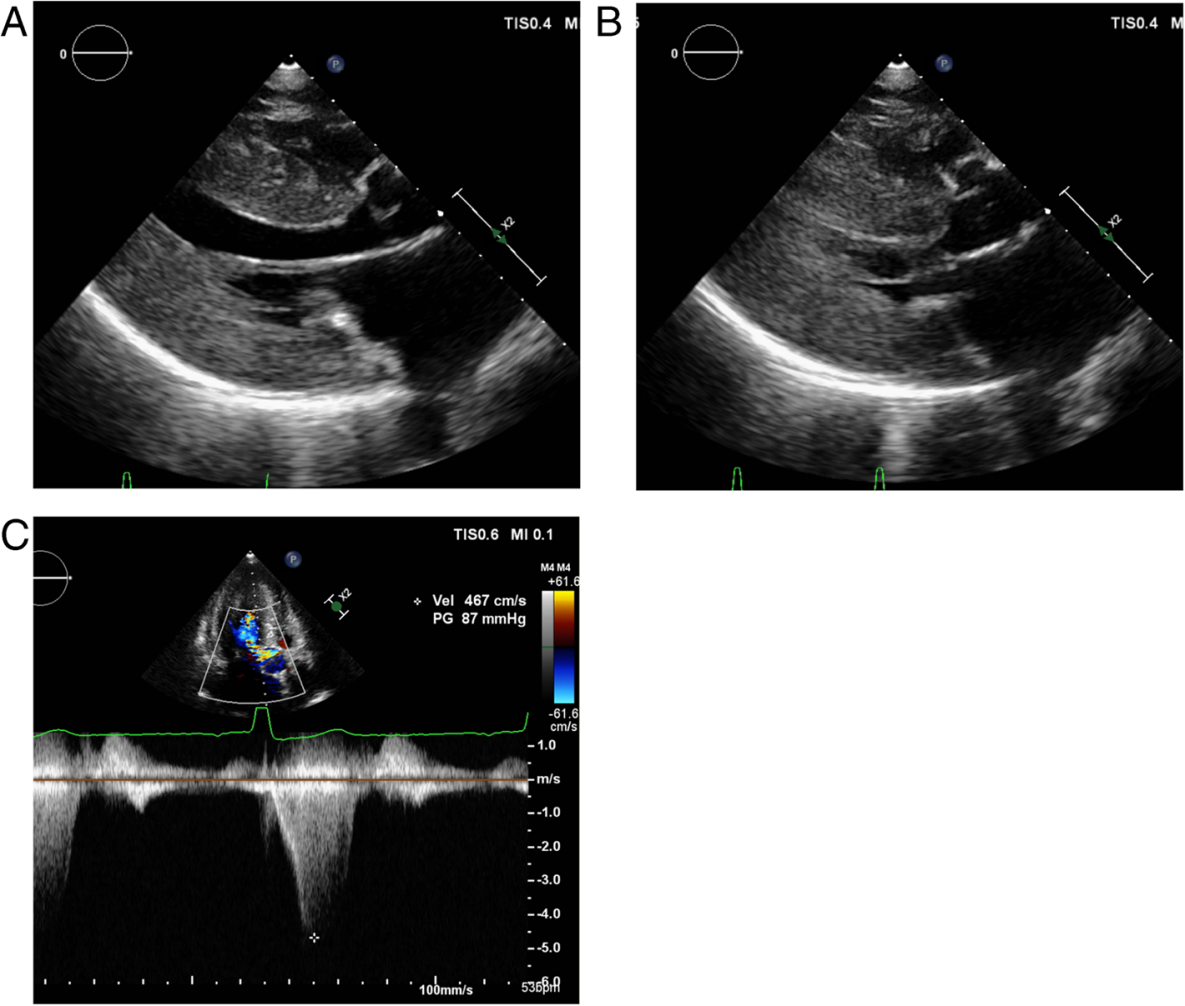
Echocardiographic findings. a, b Echocardiographic images of para-sternal long axis view of LV showing diffuse cardiac hypertrophy at diastolic (a) and systolic (b) phases. Systolic anterior motion of anterior mitral leaflet was observed. c Measurement of the pressure gradient between mid-LV and LV outflow tract
